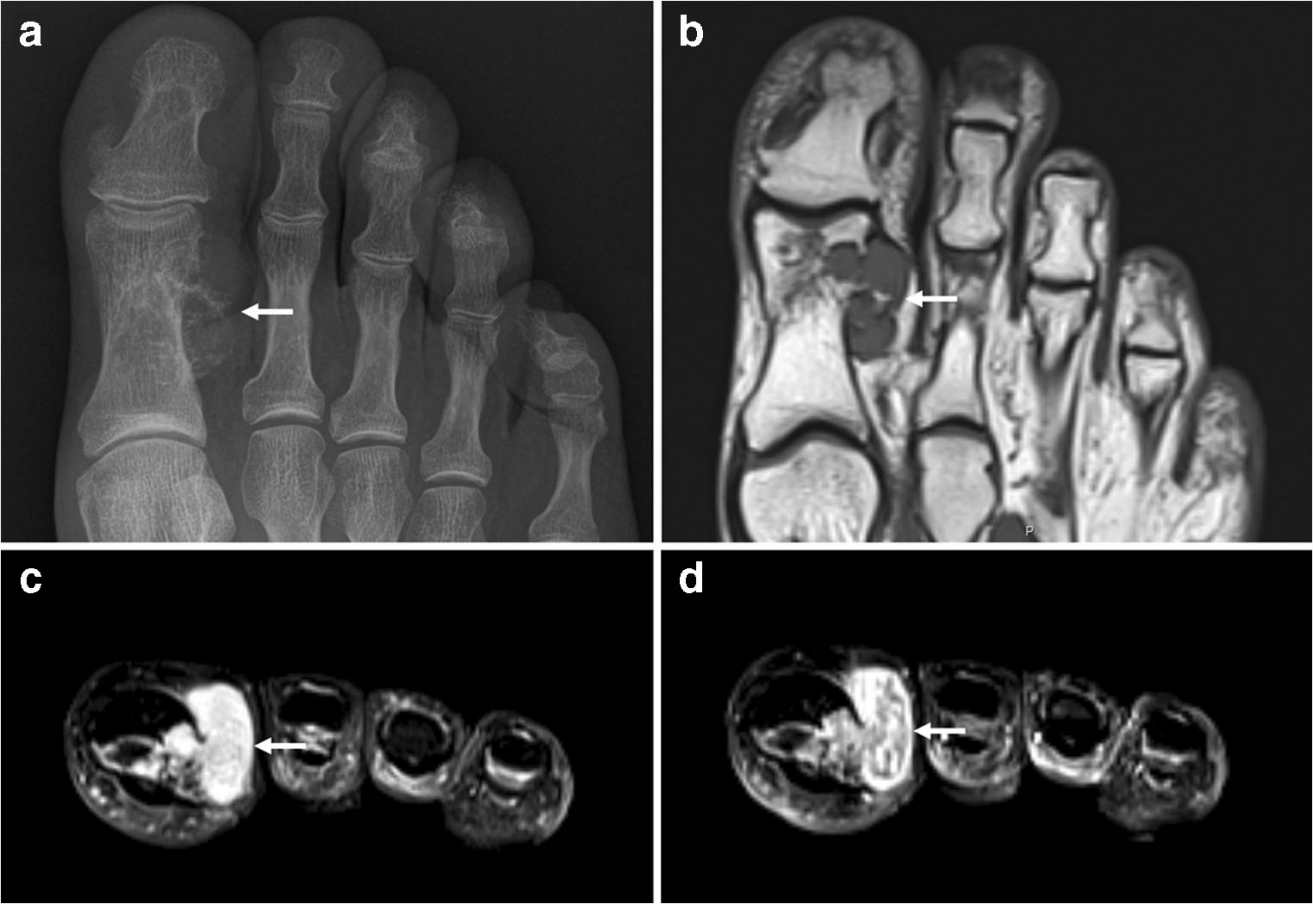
A 48-year-old man with periosteal chondroma. a Radiography shows soft tissue lesion with a calcified chondroid matrix lateral to the proximal phalanx of the first digit. b–d Axial T1-weighted image (b), coronal proton-density-weighted image with fat suppression (c), and coronal postgadolinium T1-weighted image with fat suppression (d) show pressure erosion of neighbouring bone without associated medullary bone. The lesion also displaces the flexor hallucis longus tendon. There is no soft tissue edema. The lesion shows a septal enhancement pattern (d)
